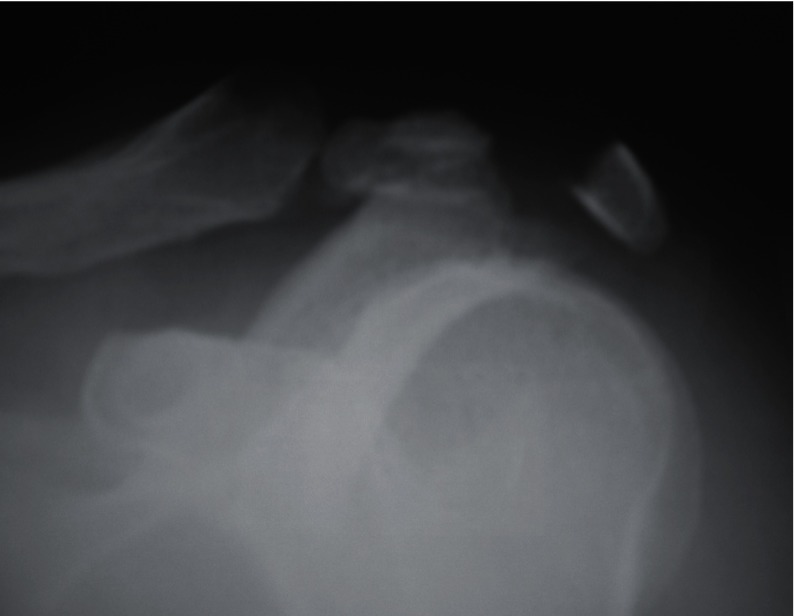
Anteroposterior Radiograph of the Shoulder Showing Displaced Fracture of the Acromial Base
